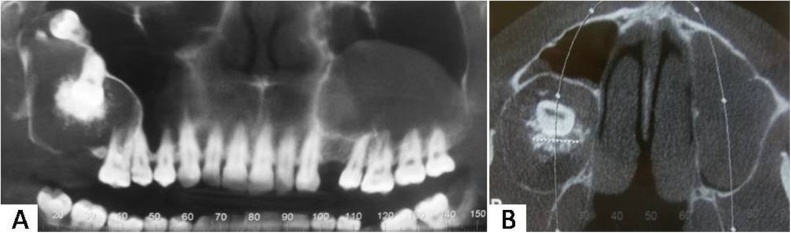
Coronal (A) and axial (B) computed tomography images.
